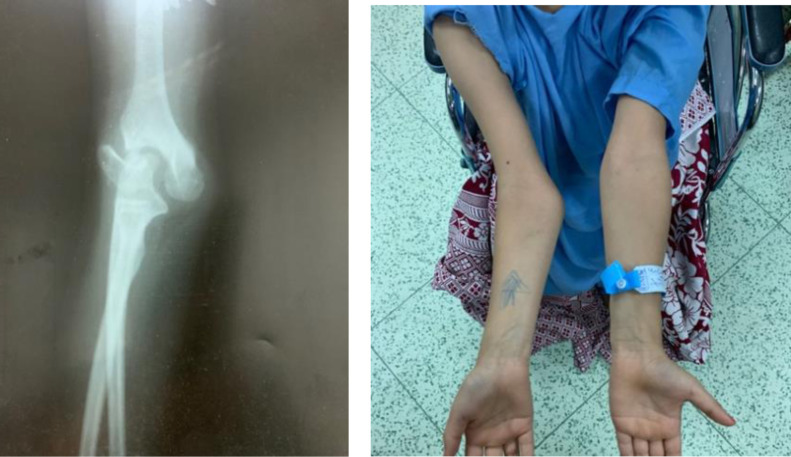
Preoperative X-ray and clinical photo of case no. (3).
